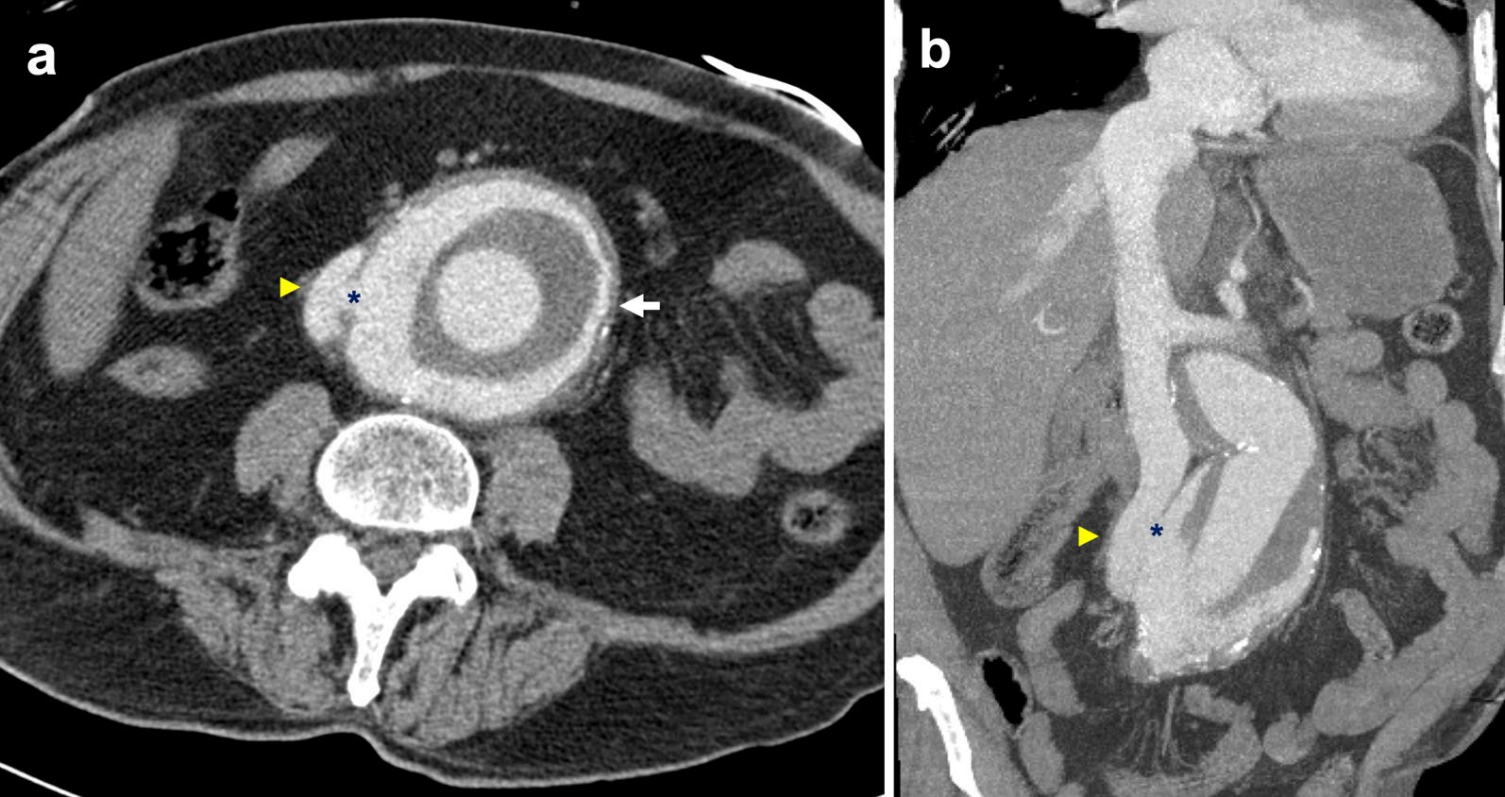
Aortocaval fistula complicating abdominal aortic aneursym. a Axial and b coronal contrast-enhanced CT images of a fistula (asterisk) between the IVC (arrowhead) and an abdominal aortic aneurysm (arrow), with similar contrast opacification of the aneurysm sac and the IVC in the arterial phase. The abdominal aortic aneurysm contains a combination of contrast opacification and non-enhancing thrombus
